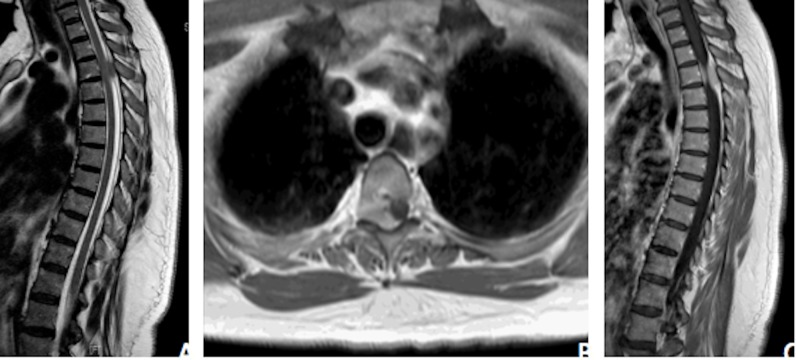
Preoperative Thoracic Spine MRI A. Preoperative sagittal T2 MRI demonstrating a thoracic lesion at T3 with resultant moderate compression of the spinal cord. B. Preoperative axial T1 post-gadolinium MRI demonstrating extension of the lesion into the right T3-4 neural foramina and causing leftward displacement of the cord. C. Preoperative sagittal T1 post-gadolinium MRI demonstrating homogenous enhancement of the thoracic lesion.
